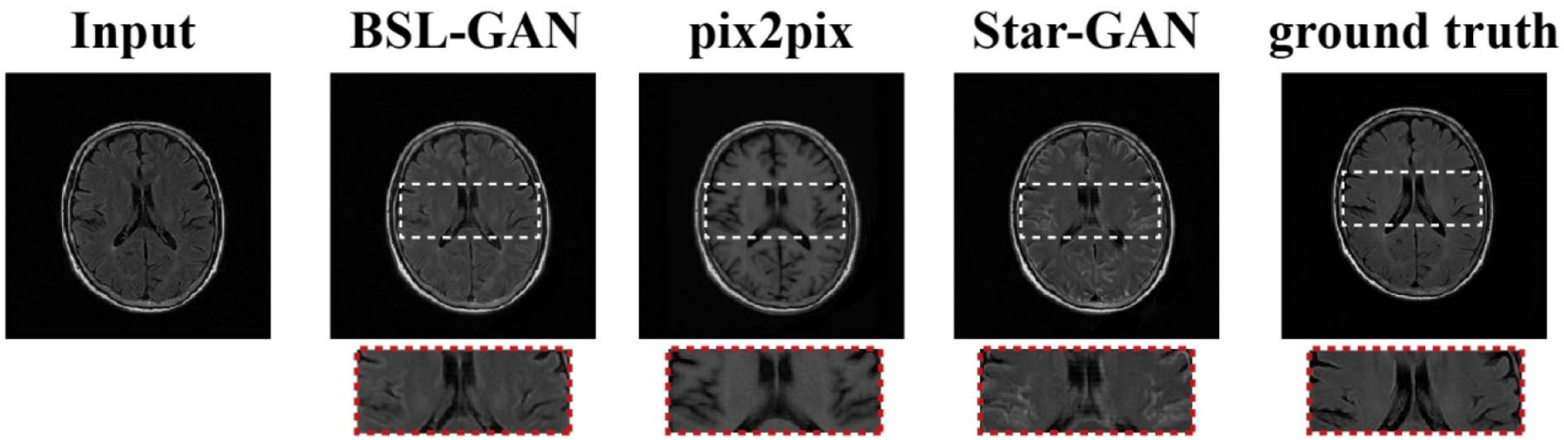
Task of 1.5T MRI to 3T MRI.
